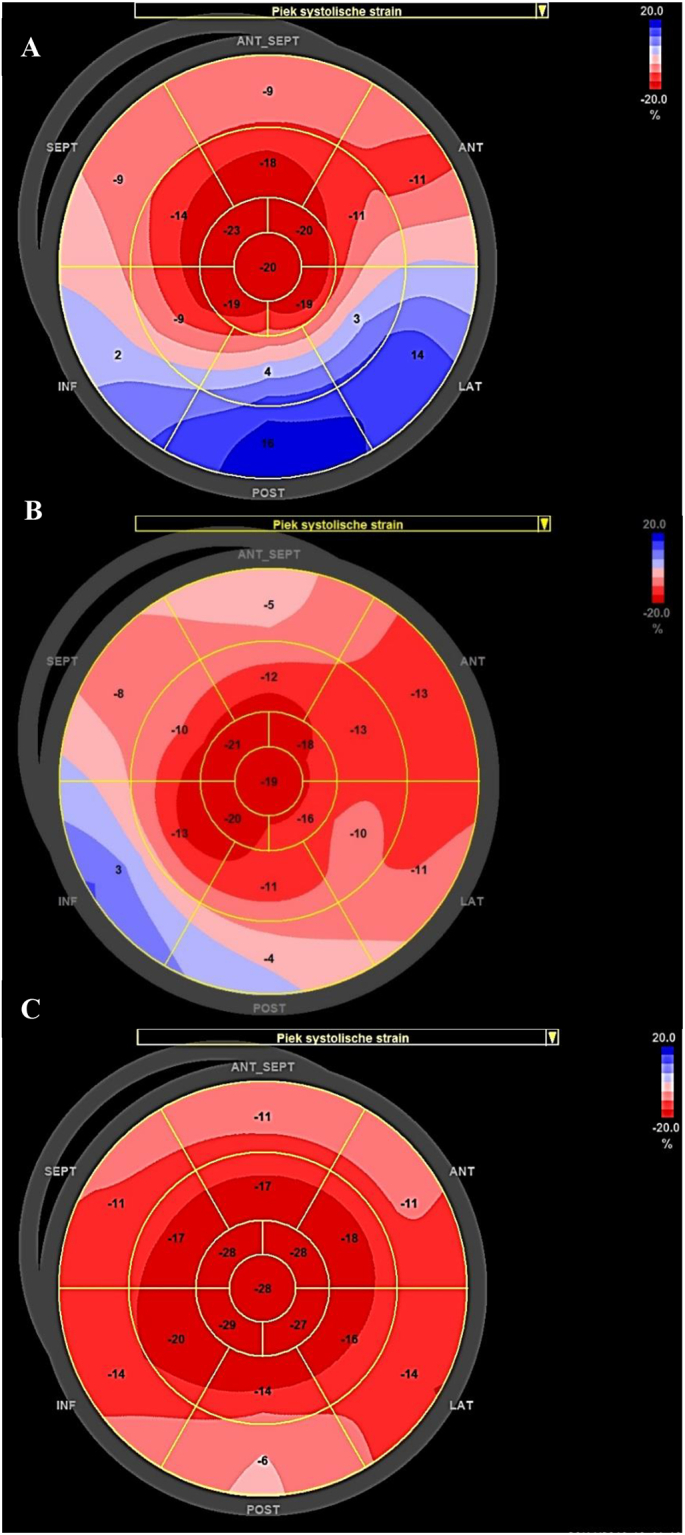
Speckle tracking echocardiography. A. Bull's-eye representation of longitudinal left ventricular strain obtained in the standard apical 2-, 3- and 4-chamber views (17 myocardial LV segments), showing apical sparing. Apical sparing is a pattern of regional (segmental) differences in longitudinal strain in which LS in the basal (outer circle) and midventricular segments of the LV is more severely impaired (blue and light red) compared with the strain values in the apical segments (inner circle, dark red segments). The longitudinal strain values of the apical segments are normal. B. Bull's-eye plot of longitudinal left ventricular strain 3 weeks after initiation of treatment. Although there is apical sparing there is global improvement of longitudinal strain values, particularly in de basal and midventricular segments. Again there is preserved longitudinal strain in the apical segments. C. Bull's-eye plot of longitudinal left ventricular strain 2 months after initiation of treatment. The apical sparing pattern is no longer present. There is global recovery of longitudinal strain in the whole left ventricle, except for 3 segments with mild impaired longitudinal strain (light red segments; basal antero-septal segment; basal anterior segment and basal posterior segment). (For interpretation of the references to color in this figure legend, the reader is referred to the web version of this article.)
